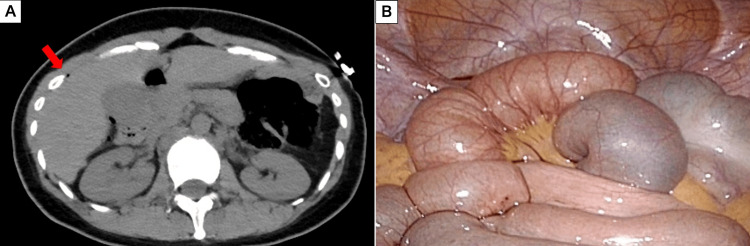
Imaging and intraoperative findings of Case 3. (A) Axial CT image demonstrating a small amount of free gas on the ventral side of the liver (red arrow). (B) A comprehensive laparoscopic exploration of the entire abdominal cavity revealing no evidence of hollow viscus perforation or contamination.
